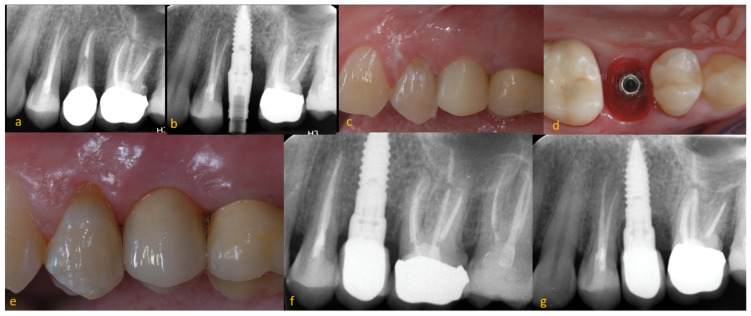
Treatment protocol applied. (a) Initial pre-extraction X-ray, (b) implant insertion, (c) immediate provisional crown, (d) peri-implant tissues, (e) final crown (4 months after implant placement), (f) X-ray of the final crown (4 months after placement implant), (g) X-ray of the final crown (24 months after implant placement).
